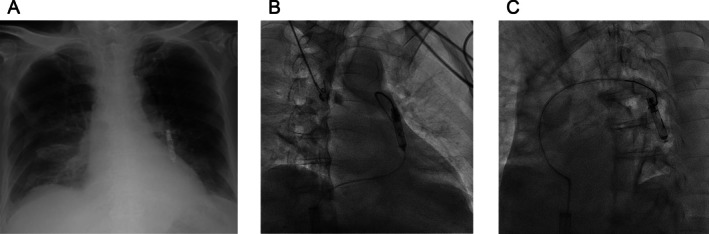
(A) Chest radiograph (posterior–anterior view) showing the dislodged leadless pacemaker located outside the heart. (B) Cine angiography image in the right anterior oblique 30° view, showing the snare catheter capturing the helical portion of the leadless pacemaker. (C) The same maneuver viewed in the left anterior oblique 50° view.
